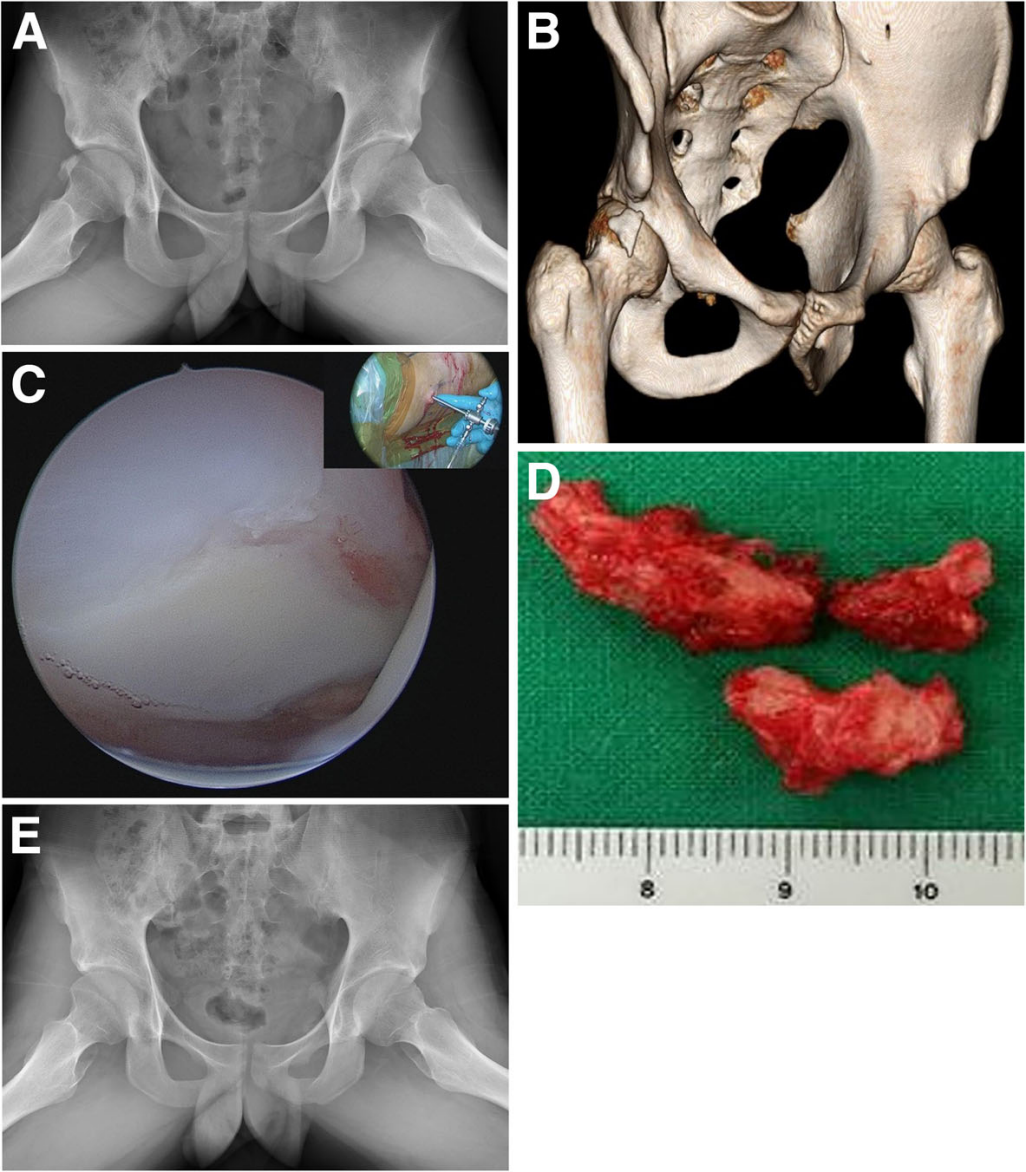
a, b Frog-leg lateral radiograph and 3D CT scan of a 19-year-old male patient, acquired 1 year after arthroscopic labral repair and acetabulofemoroplasty. Heterotopic ossification was classified as Brooker grade 2, with the largest measurement (width) being 21 mm. c, d Heterotopic ossification was located in anterolateral joint capsule and removed with arthroscopy. e Frog-leg lateral radiograph showed no evidence of heterotopic ossification at 2 year follow-up
